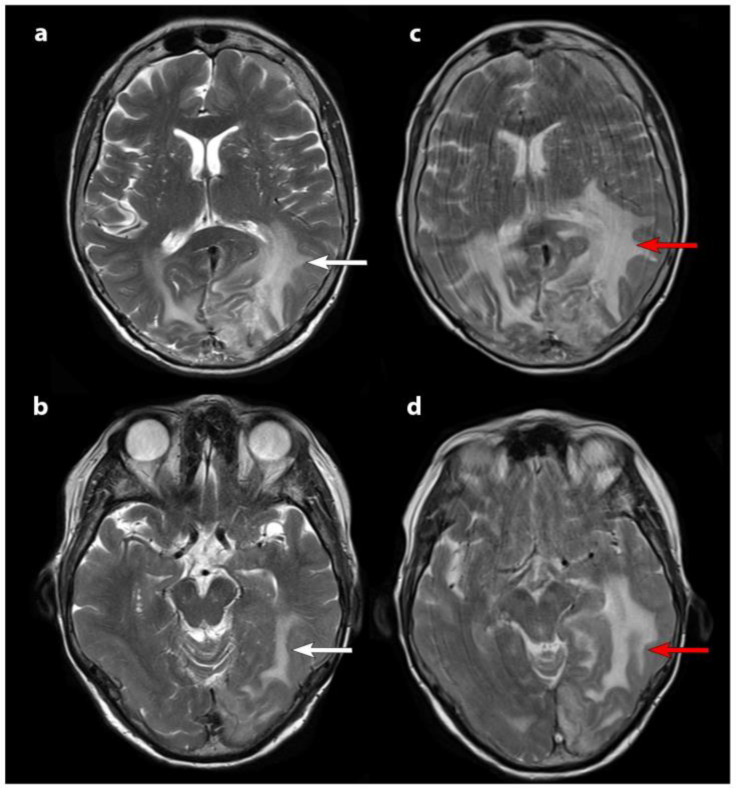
T2-weighted axial MRI scans: pre-treatment MRI images (panels (a,b)) and post-second cycle PRRT scans (panels (c,d)). White arrows indicate baseline brain edema, which worsens after the second cycle, as shown by red arrows. The increased motion artifacts in the second MRI (panels (c,d)) correspond to the patient’s clinical decline, aligning with radiological progression. Reprinted from [8] under CC BY license.
